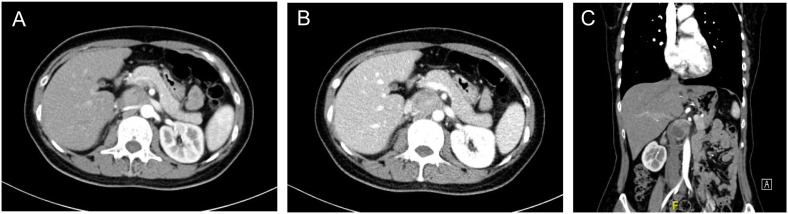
Enhanced CT of retroperitoneal PGL (A) Arterial phase of the PGL. The tumor was located behind the pancreas, pushing the right renal artery dorsally and adjacent to the abdominal aorta (B) Portal phase of the PGL. The inferior vena cava was pushed to the right and the left renal vein ran in front of the tumor. (C) Coronary position of the PGL.
